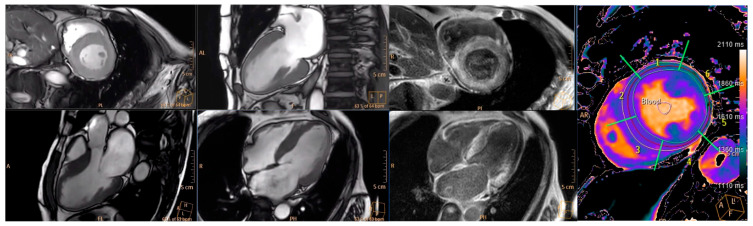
Example of cardiac magnetic resonance images showing typical pattern of ATTR-CM in one of the patients before diflunisal treatment initiation. Cine frames, contrast enhanced images and cardiac T1 mapping sequences are displayed on the left, medium and right of the figure, respectively.
